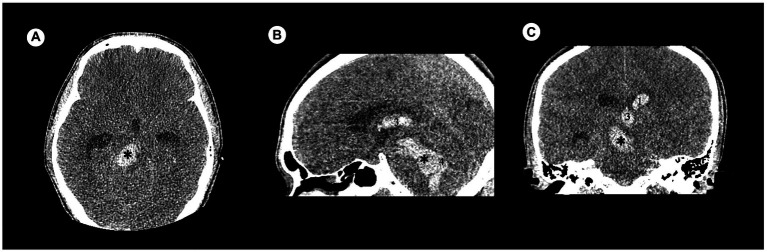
Examples of hemorrhage involving the ventricular system in patients with acute obstructive hydrocephalus detected by craniofacial computer tomography (CT) scans. (A) Transverse view: Hematoma was present in brainstem (✱). (B) Sagittal view: Hematomas were present in brainstem (✱), third ventricle (3), and fourth ventricle (4). (C) Coronary view: Hematomas were present in brainstem (✱), third ventricle (3), and lateral ventricle (1).
